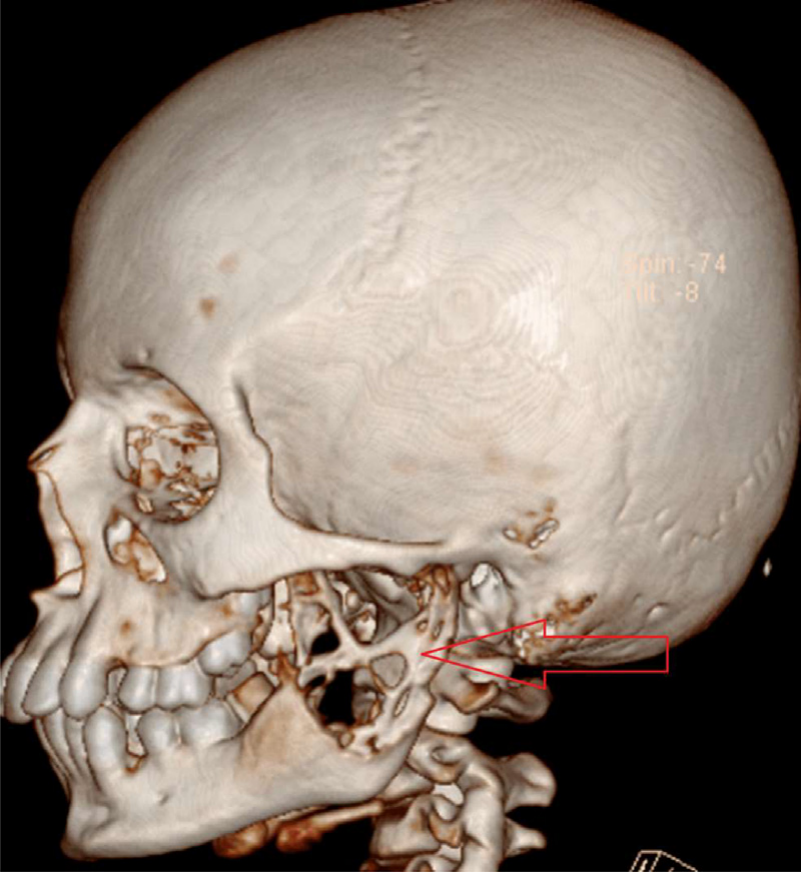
Reformatted CT scan image of the skull demonstrating expansile cystic lesion with cortical erosion in area of left hemimandible (red arrow).
